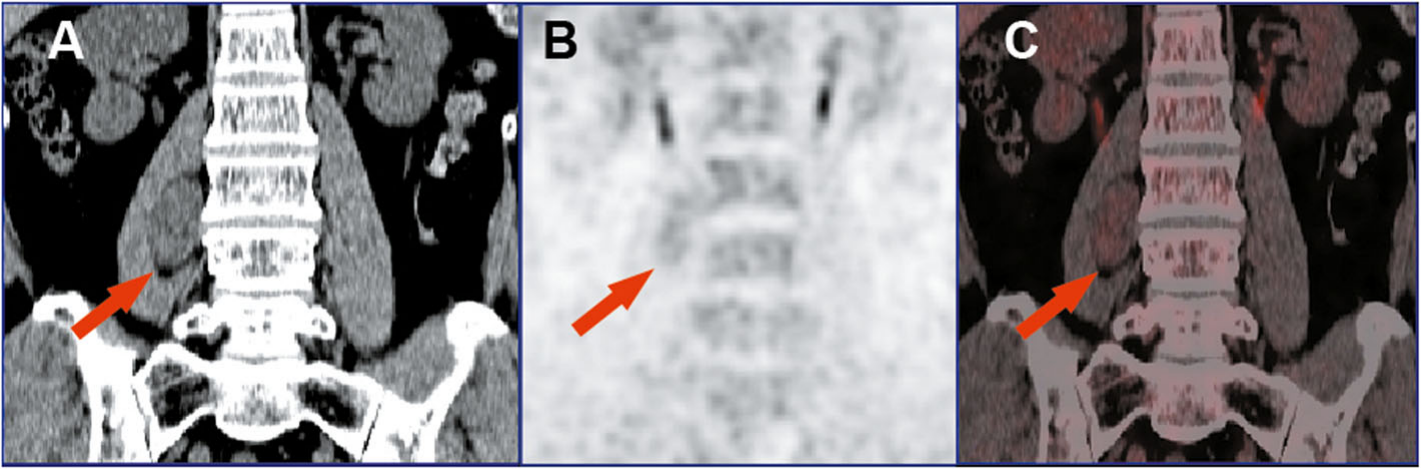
Schwannoma of the right psoas major. (a) CT- coronal plane. (b) PET- coronal plane. (c) PET/CT fusion image. As indicated by the red arrow, the density of the mass was slightly lower than that of the adjacent muscle tissue. Moreover, the uptake of ^18^F-FDG increased moderately and homogeneously. Tumor size = 2.5 cm, SUVmax = 3, HF = 0.36, TLG = 25.5, MTV = 15 cm^3^, and *P* value = 0.21
